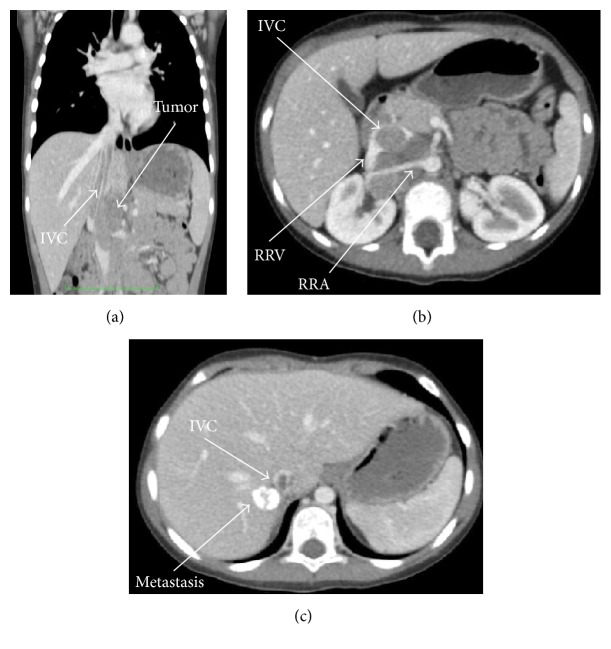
Computed tomographic scanning showing (a) incomplete obstruction of inferior vena cava (IVC) by tumor mass (b) incorporating the right renal artery (RRA) and the right renal vein (RRV). Abdominal imaging revealed a calcified solitary hepatic lesion (c).
